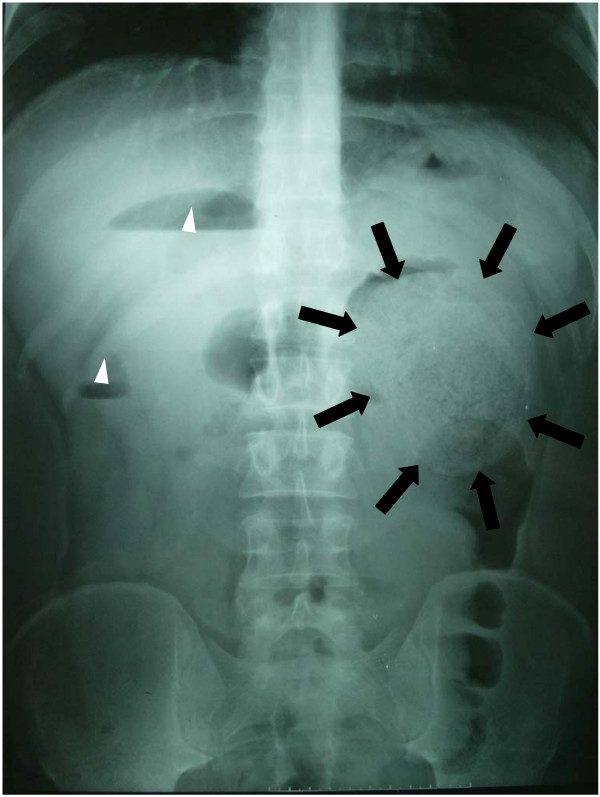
Abdominal Plain Film. Black arrow showed a large mass (the fecal calculus) with clear border on the left upper quarter of the abdomen. White arrow head show two vapor-liquid levels.
